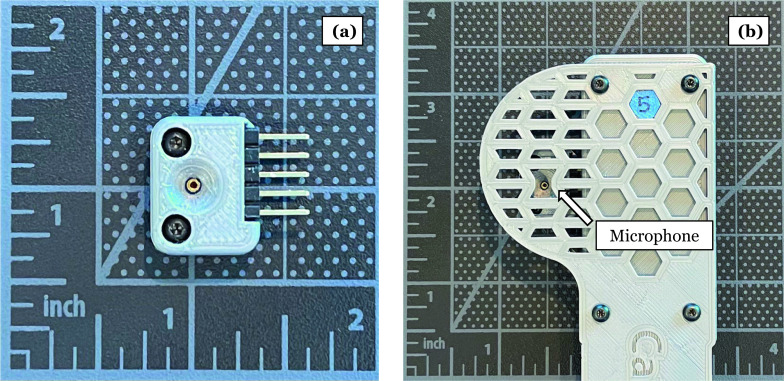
A microphone (a) was embedded along with the on-board camera controller (b) to trigger the camera when the audible alert from the radiography machine was emitted during radiograph acquisition
